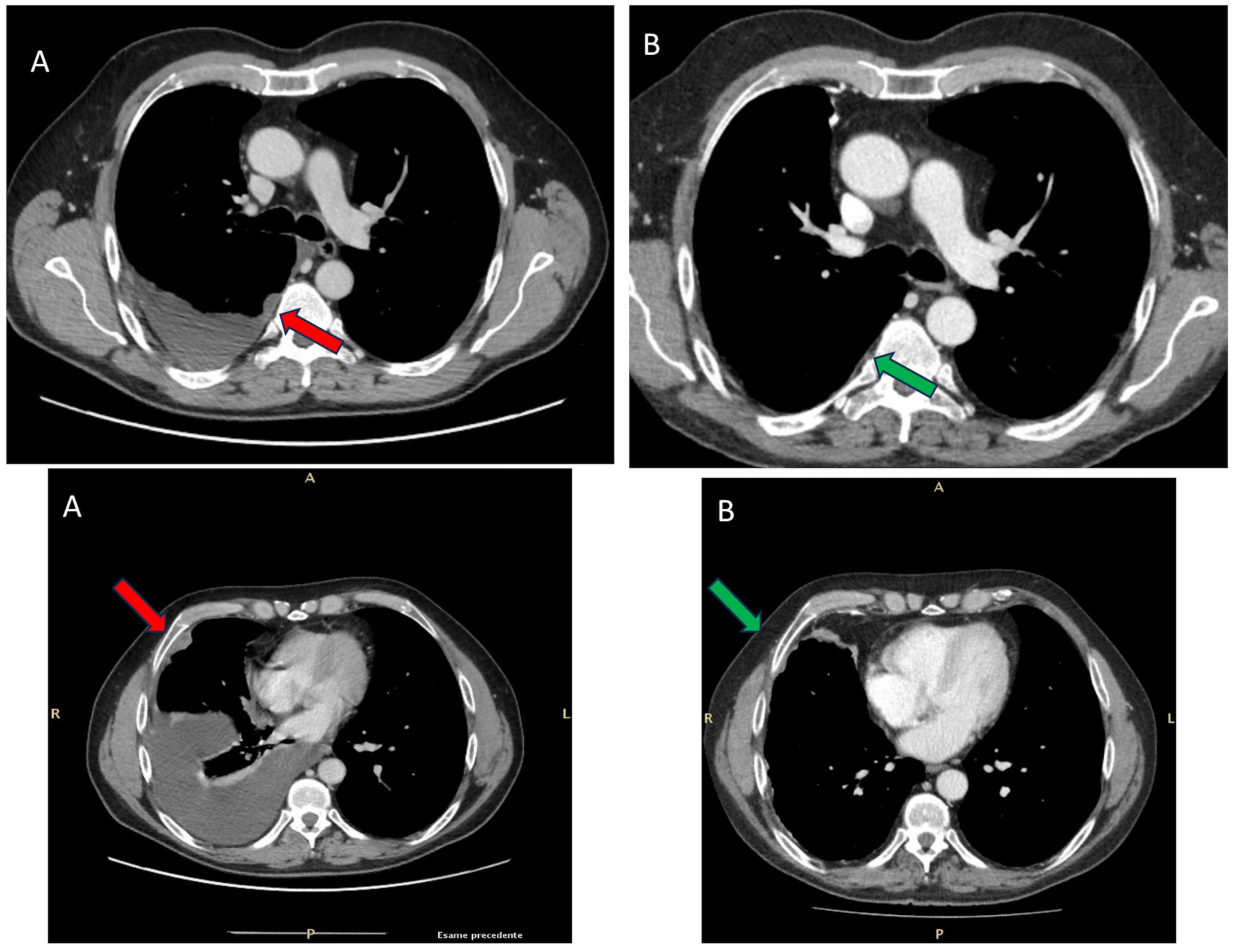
Chest computed tomography scan of the patient before (A) and after four cycles of immunotherapy (nivolumab and ipilimumab) (B). In image A, the red arrow indicates the significant pleural thickening, while in B, the green arrow highlights the substantial reduction in the disease burden.
